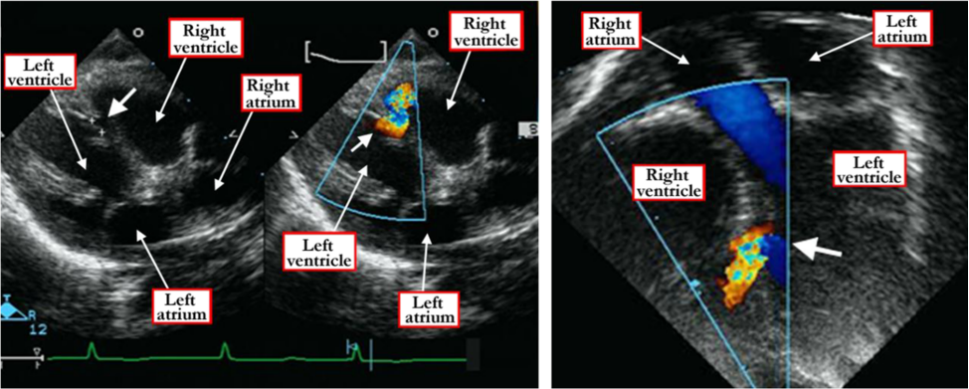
The cross-sectional echocardiogram, taken in slightly off axis four chamber projections, shows an apical muscular ventricular septal defect (white arrow) in the left hand panel of Figure
